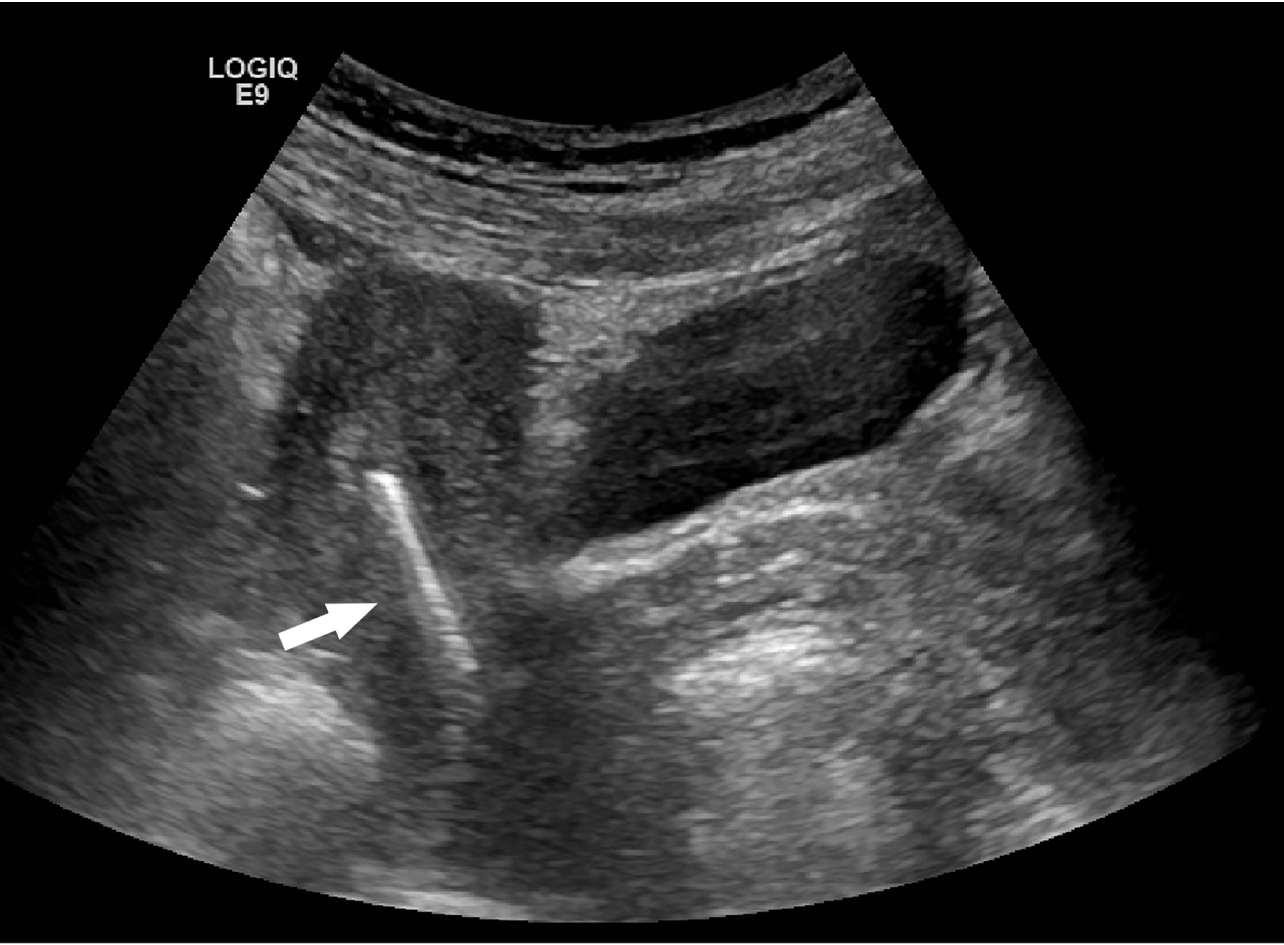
Transvaginal, sagittal sonogram demonstrating an intrauterine device in the left horn (bright white bar, see arrow).
